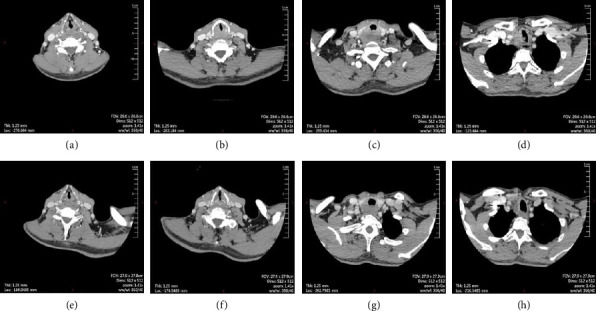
Preoperative enhanced computed tomography axial images of the neck. (a–d) The images showed that the patient had abnormally enhanced right lobe before the start of targeted therapy, indicating TC, tumor lesions range of about 30 × 43 × 50 cm, the left lobe of the thyroid gland, ring cartilage, trachea, and subglottic areas had been invaded, and bilateral multiple cervical lymphadenectasis. (e–h) The images showed that the tumor lesion area of the patient had been reduced to 34 × 36 × 48 cm after 1 month of targeted therapy, and the density was reduced compared with the previous time.
